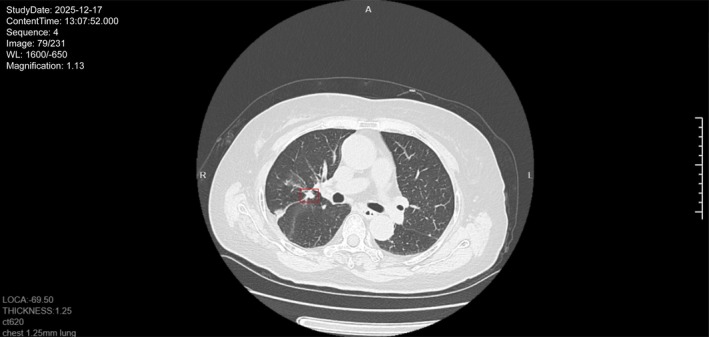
Follow‐up chest computed tomography performed on December 17, 2025, showed continued improvement, with further reduction of the right lung mass to 11 × 7 mm.
